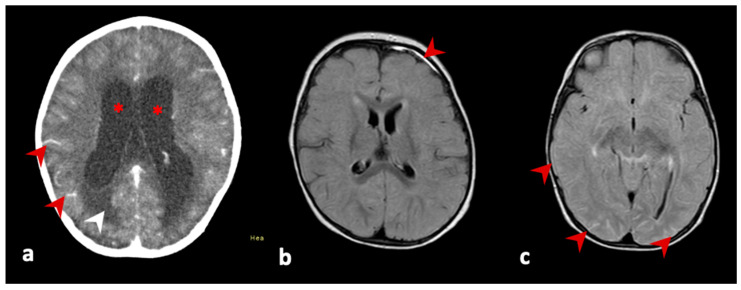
(a) Brain nuclear magnetic resonance (NMR) imaging of patient one. Dilatation of the ventricular system (asterisks) with signs of ependymal transudation (white arrowhead), and abnormal diffuse leptomeningeal enhancement (red arrow heads) in the image acquired with pre-contrast T1-weighted images. (b) Patient two’s radiologic findings. Focal pachymeningeal enhancement in the left frontal region (arrow) in the image acquired using fluid-attenuated inversion-recovery (FLAIR). (c) Patient three’s radiologic findings. Arrows indicate the presence of diffuse leptomeningeal enhancement in the image acquired using fluid-attenuated inversion-recovery (FLAIR).
